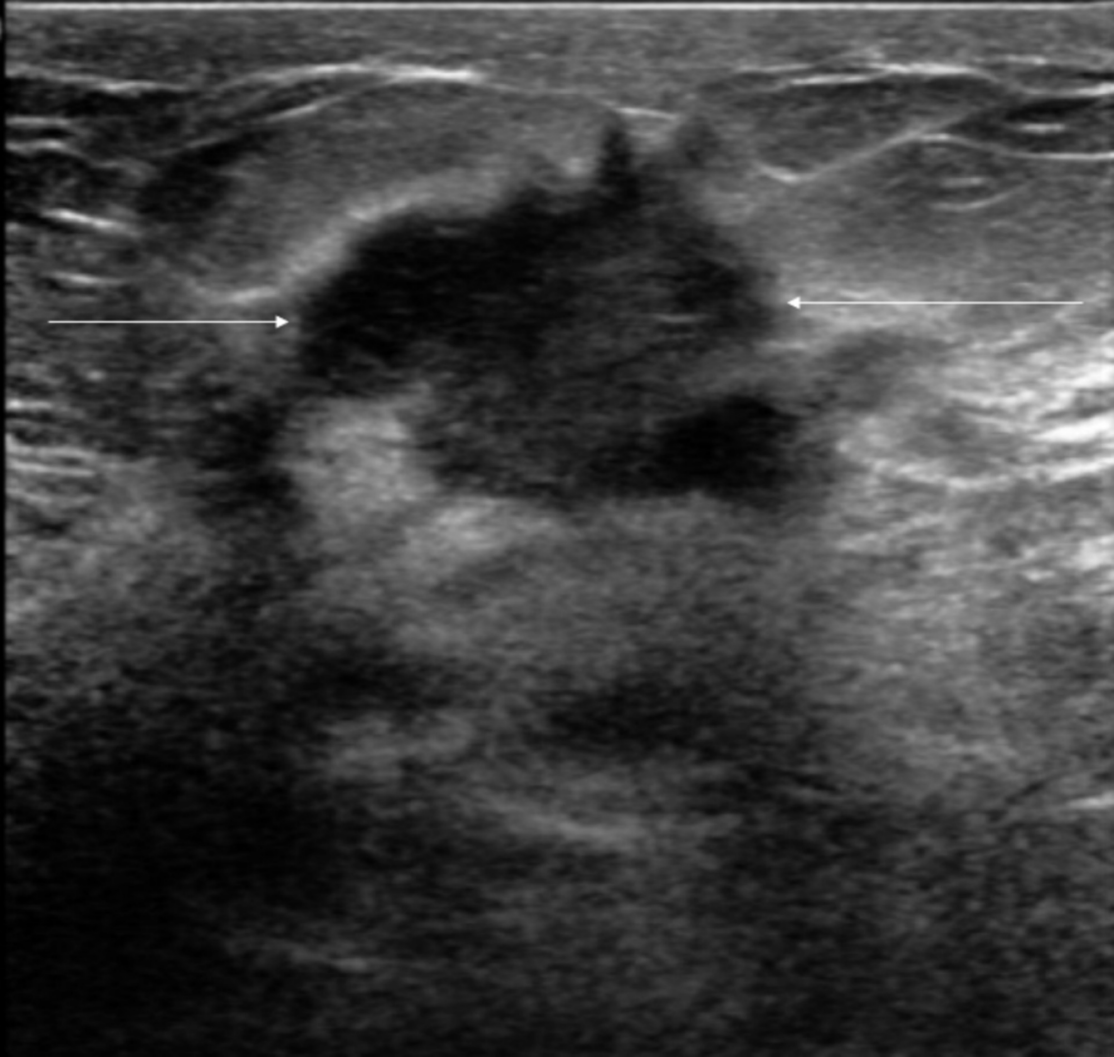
Ultrasound of the right breast showing the hypoechoic region
